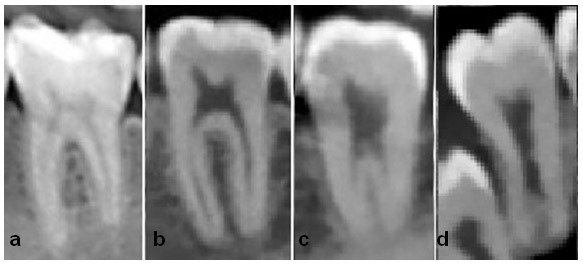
Radiographic views of taurodontic teeth a: Normal molar, b: Hypotaurodontism, c: Mesotaurodontism, d: Hypertaurodontism
